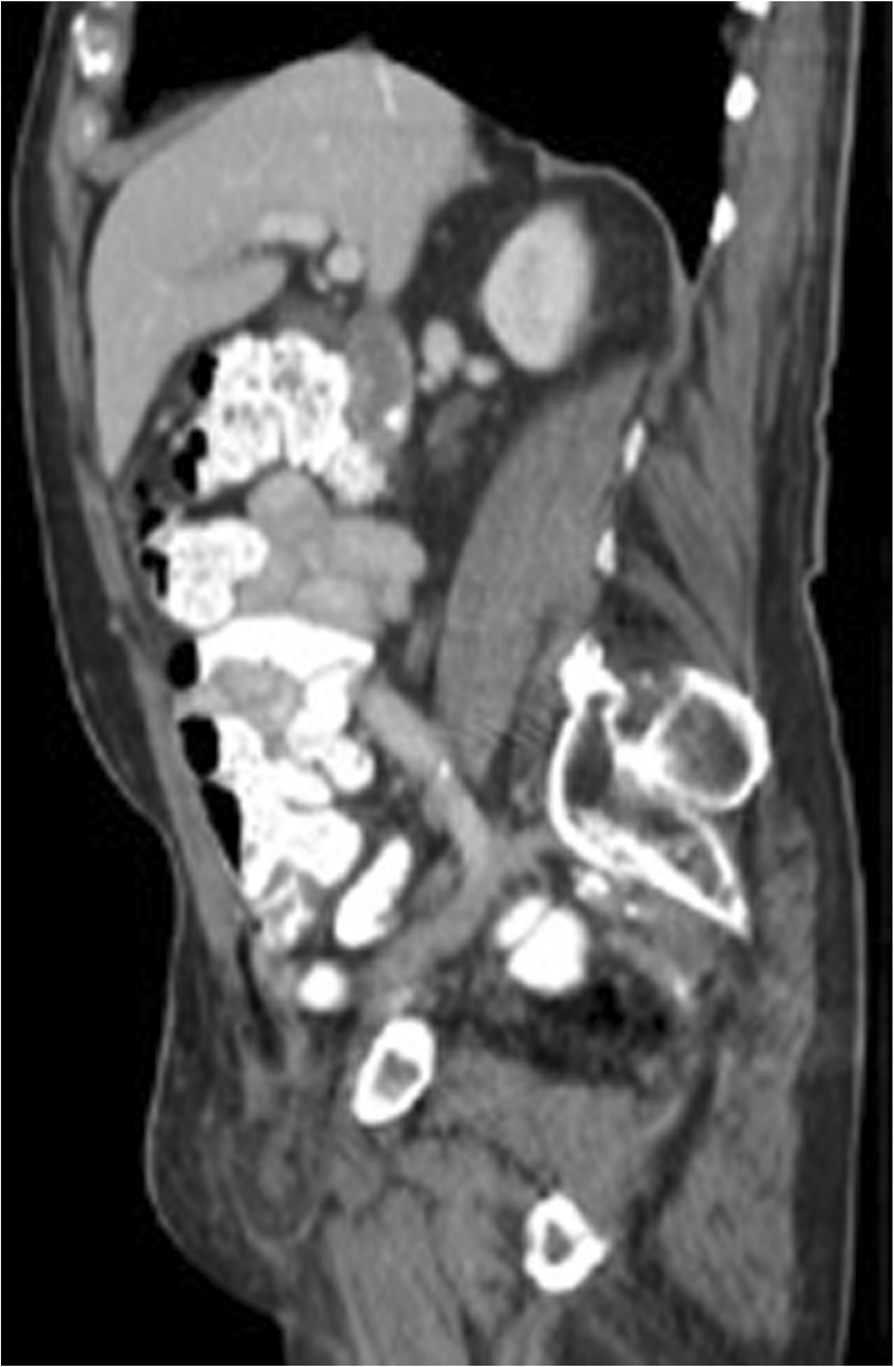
Computed tomography sagittal view of the appendix and omentum within the hernia
